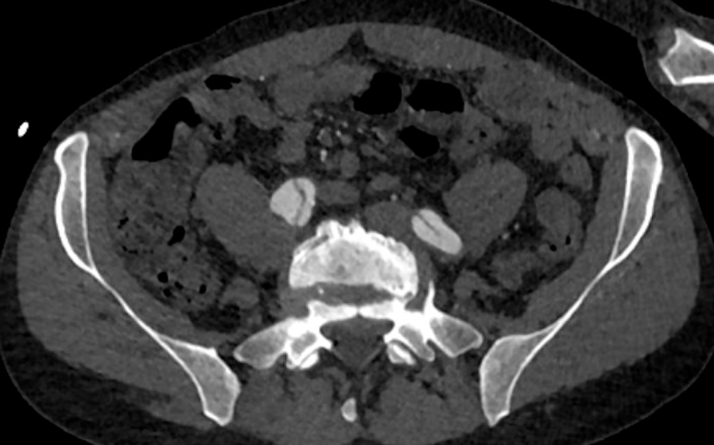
Axial Computed Tomography Demonstrating the Extent of Acute Dissection to the Iliac Arteries
